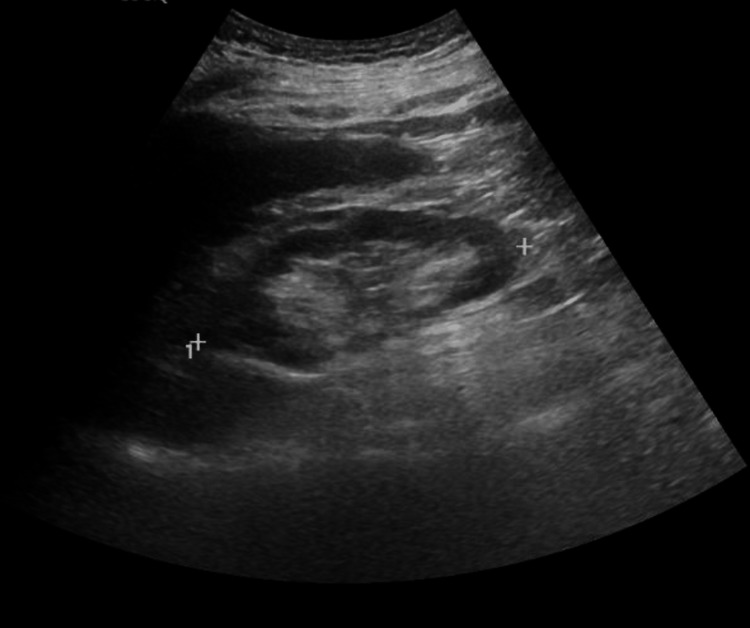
Ultrasound of the right kidney. Grayscale ultrasound image of the right kidney demonstrating grade II increased echogenicity.
